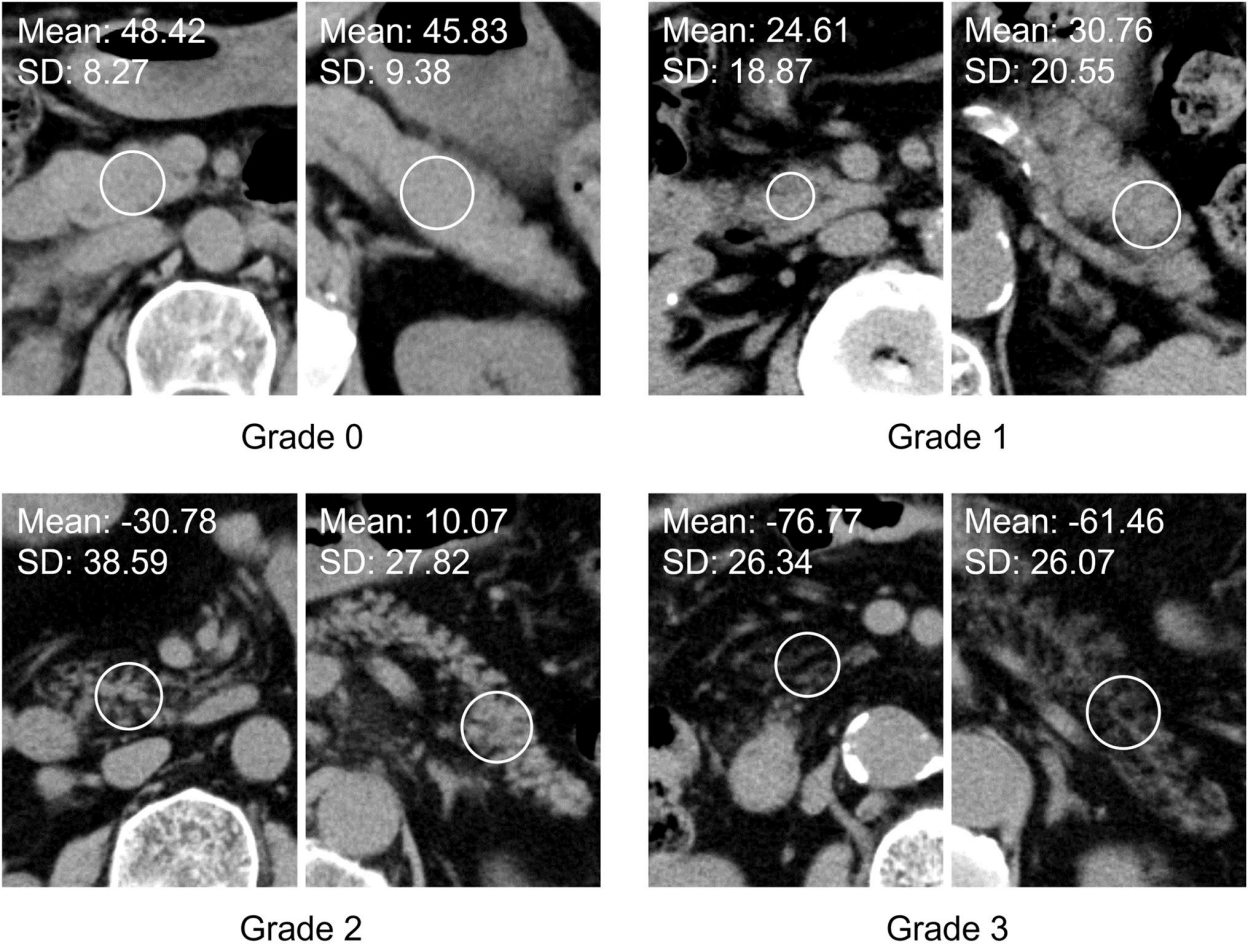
The method of ROI assignment for the CT attenuation-based assessment on pancreas head (left) and tail (right) on the same images of Fig. 1. Mean and standard deviation of CT values of each ROI are shown
